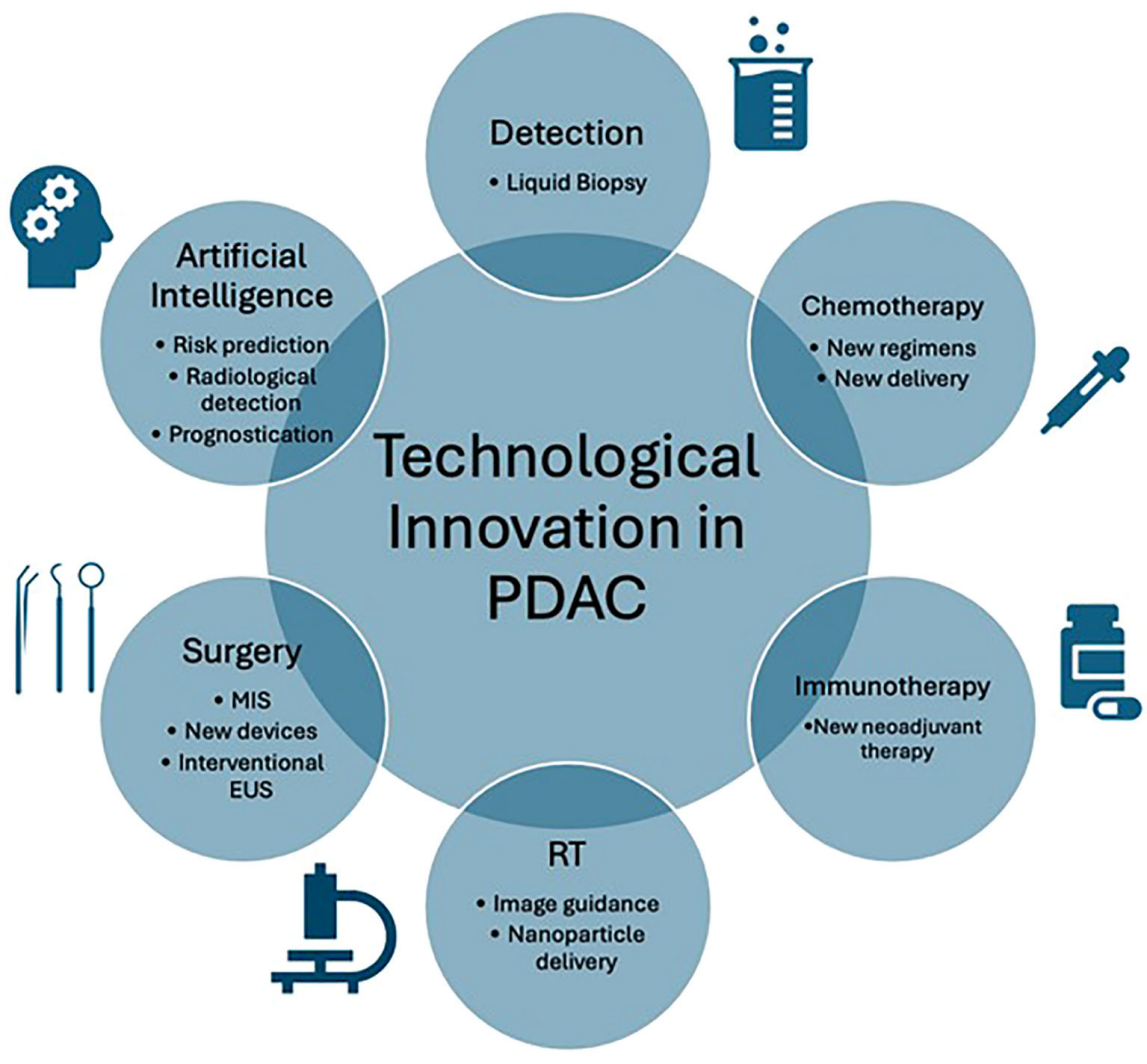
Graphical representation of technological innovation in pancreatic ductal adenocarcinoma (PDAC). EUS, Endoscopic ultrasound; MIS, Minimally invasive surgery; RT, Radiotherapy.
